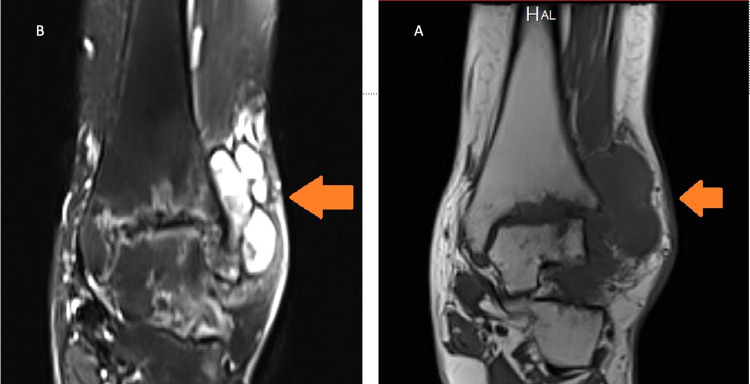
(A) T1- and (B) T2-weighted coronal view MRI of the left ankle showing multinodular mass and low signal intensities indicating that hemosiderin deposits were diagnostic of diffuse pigmented villonodular synovitis
